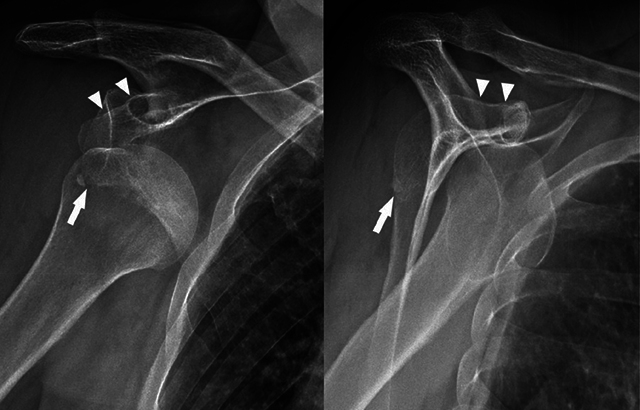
Anteroposterior and trans-scapular Y radiographs of the right shoulder demonstrating anterior subcoracoid dislocation of the humeral head (arrowheads) and displaced inferior glenoid rim fracture (arrows).
